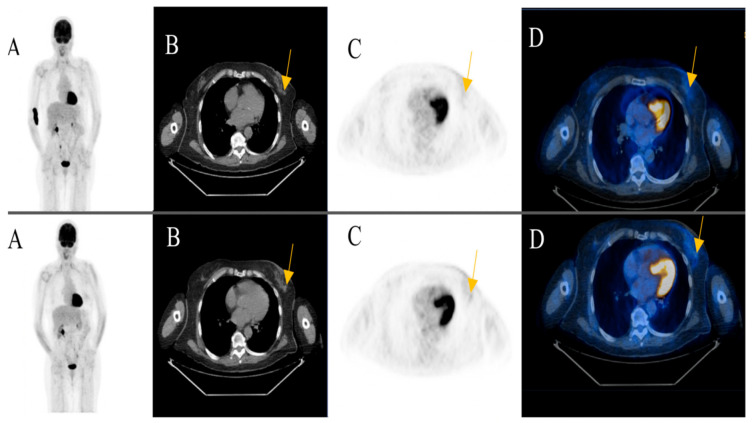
An 81-year-old female patient initially with metastatic cutaneous melanoma stage IV (pT2a N3 M1b). (A) Maximum intensity projection (MIP); (B) Computed Tomography (CT) axial of chest; (C) Positron Emission Tomography( PET) axial of chest; (D) Fused images PET/CT axial of chest. First row: FDG-PET/CT performed for surveillance after treatment without evidence of metabolically active recurrence, nodal or distant metastasis. Contamination at injection site cubital on the right upper extremity. Second row: Six months later, a morphologic and metabolic progredient subcutaneous nodule in the left breast was seen (yellow arrow). An ultrasound-guided biopsy was subsequently recommended for further evaluation. The biopsy revealed an invasive ductal breast cancer.
